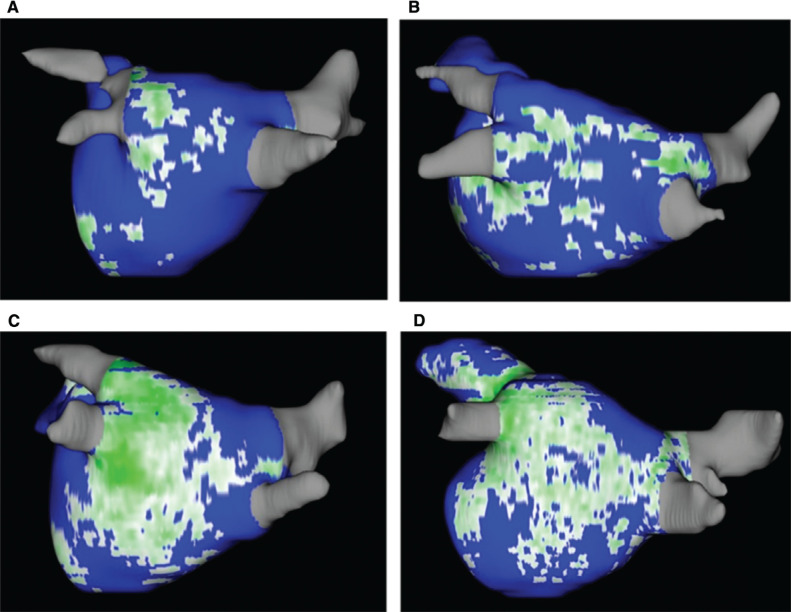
Cardiac magnetic resonance imaging of the left atrium with postprocessing of late gadolinium enhancement sequences depicting a left atrial scar. The scar is quantified and patients can be categorized by their degree of fibrosis. A: Utah class I—5.1% fibrosis. B: Utah class II—18.2% fibrosis. C: Utah class III—24.5% fibrosis. D: Utah class III—35.4% fibrosis.
